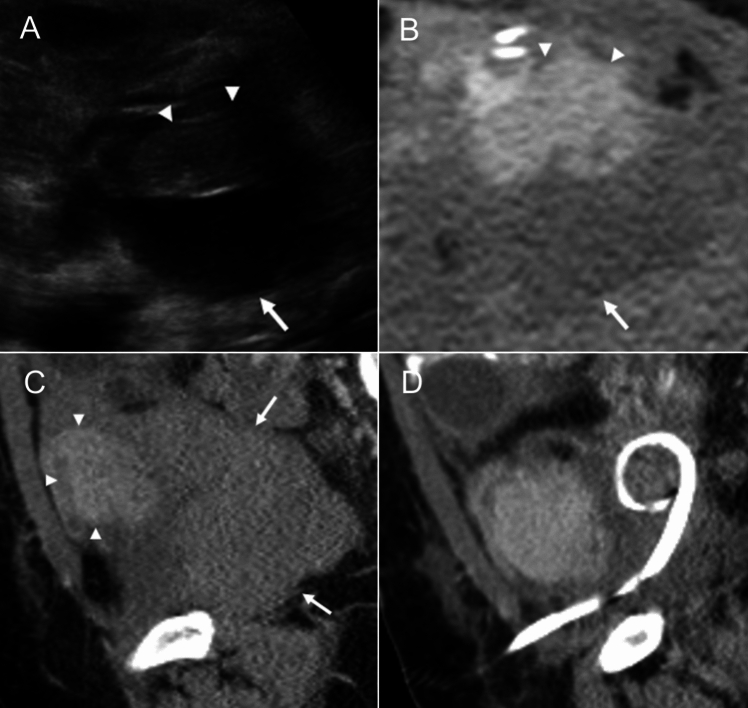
Peripancreatic fluid collection. A 31-year-old male with a history of type 1 diabetes mellitus and nephropathy underwent pancreas after kidney transplantation. Grayscale ultrasound (A) on postoperative day 14 demonstrates anechoic simple fluid collection (arrow) adjacent to the pancreas graft (arrowhead). Contrast-enhanced computed tomography (CT) (B-C) shows hypodense to isodense and non-enhancing fluid collection (arrow) adjacent to the pancreas graft (arrowhead). Pre-drainage CT (C) demonstrates the extent of the fluid collection, and post-drainage CT (D) shows a significant decrease in the size of the fluid collection following percutaneous drainage tube placement
